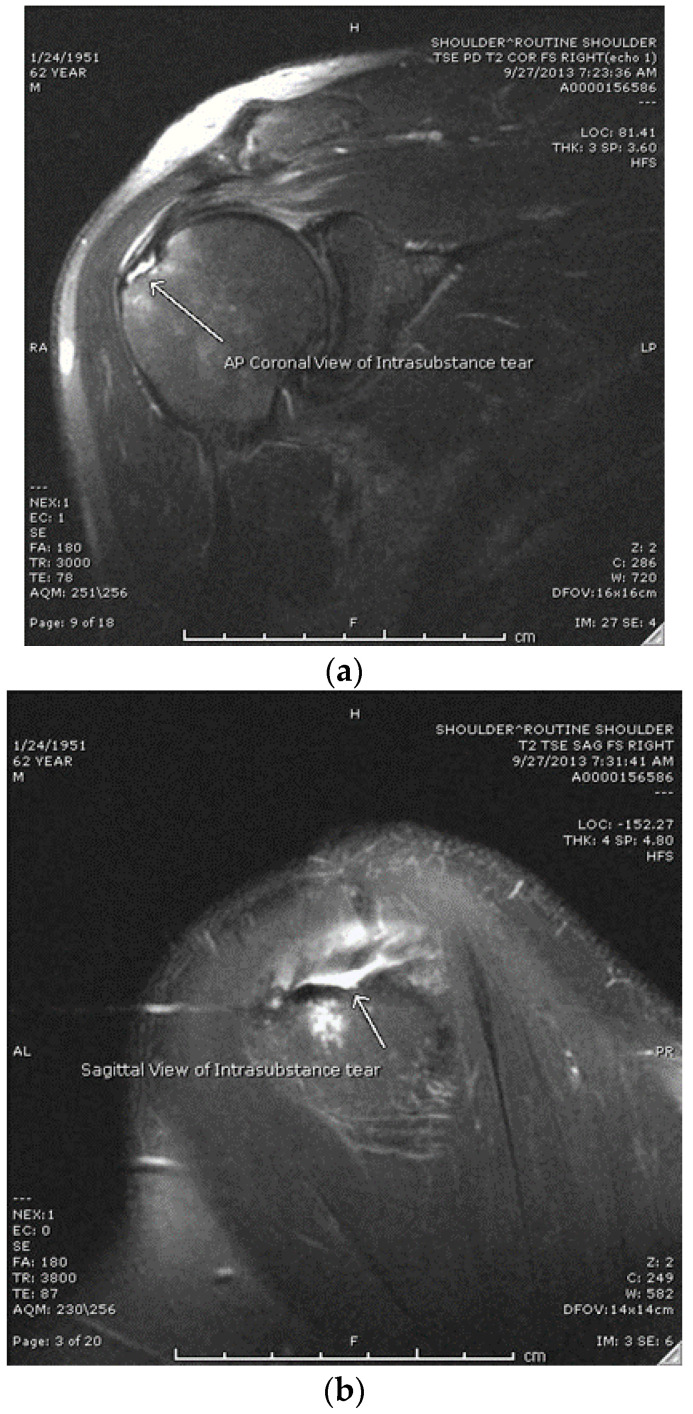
(a) Coronal T2 image from MRI showing an intrasubstance rotator cuff tear. (b) Sagittal T2 image from MRI showing an intrasubstance rotator cuff tear.
